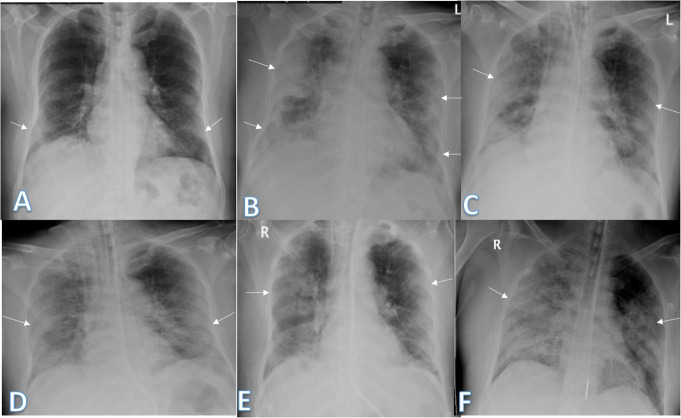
59-year male with positive COVID-19 virus. Initial X-ray (a) showed small peripheral right and left lower zonal air space consolidation opacities (arrows) with TSS as 2. On the 3rd day (b), air space consolidation opacities increased extended to the periphery of the upper and middle zones of both lungs (arrows) with TSS as 5 (3 on RT side and 2 on left side). The patient deteriorated and was intubated. On the 5th day (c), 6th day (d), 9th day (e) and 11th day (f), there was confluent consolidation (arrows) more on the right lung with severity score 4 on the right side and 3 on the left side, so TSS was 7. The patient died on the 11th day
